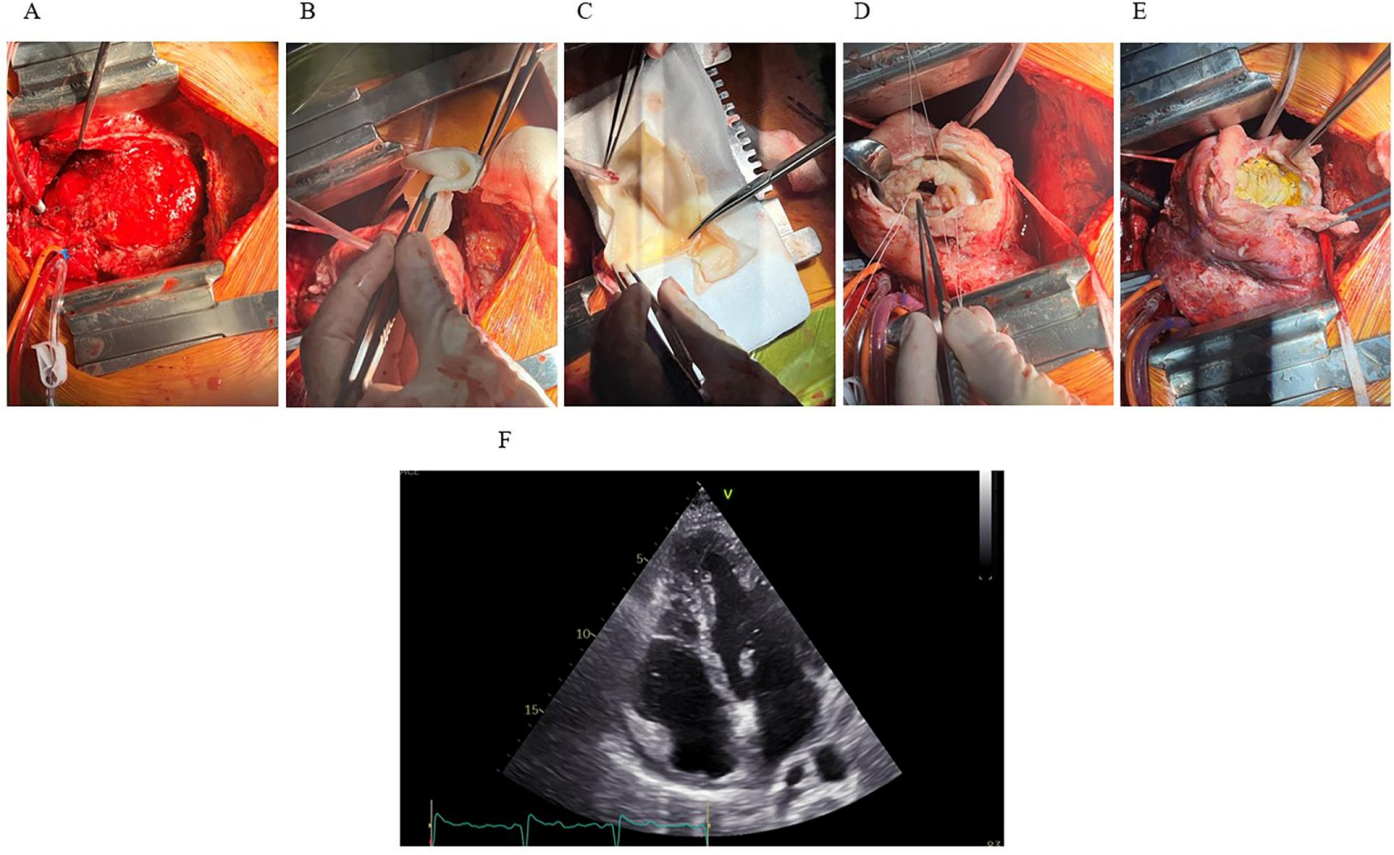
The Dor procedure and outcome. (A) Median sternotomy reveals the heart exposed, showing extensive hematoma and inflammation involving the inferoapical myocardial region. (B,C) A synthetic patch for reconstruction of the LV geometry. (D) LV cavity exposed following resection of infarcted and necrotic myocardium. Sutures placed around the cavity for patch attachment. (E) Final positioning and fixation of the synthetic patch. (F) Postoperative TTE (apical four-chamber view) demonstrates successful resolution of the IDH and intracavitary thrombus, with only residual apical hypokinesis.
